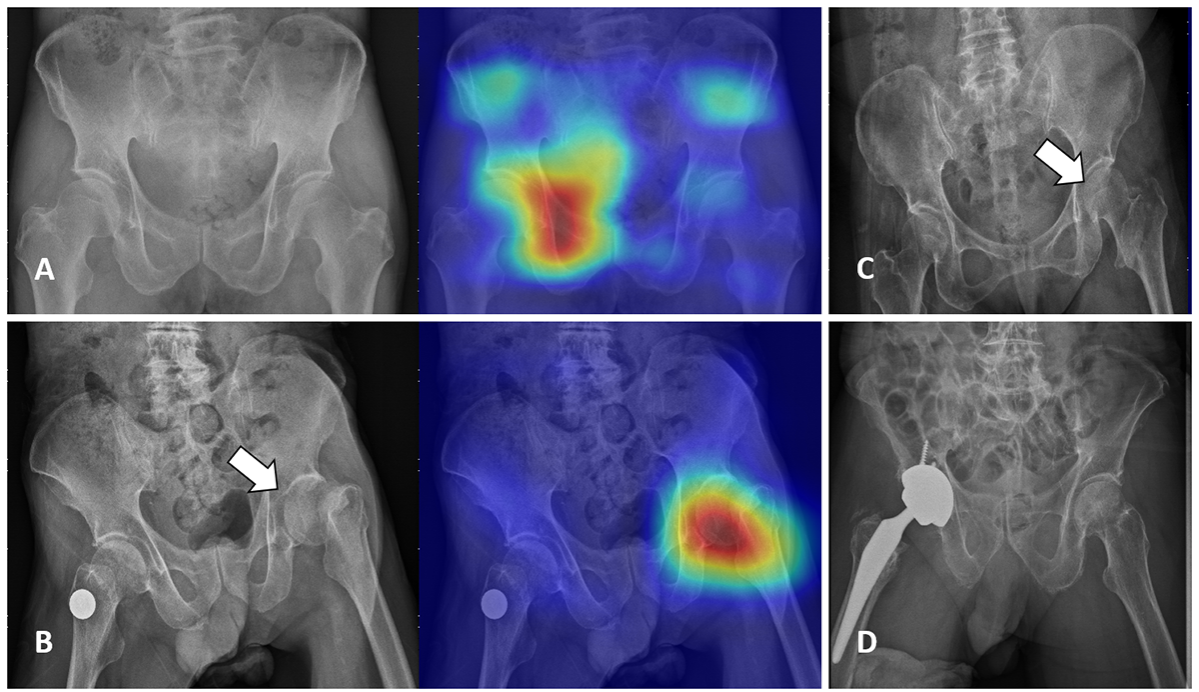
Examples of inconsistencies between the participants and the algorithm. (A) A pelvic x-ray (PXR) without hip fracture that was overdiagnosed by the algorithm. No participant overdiagnosed in the physician-alone test, and only 1 (2.9%) participant overdiagnosed in the human-algorithm integration (HAI) test. (B) A PXR with left hip fracture. In the physician-alone test, 12 (35.3%) participants missed this fracture. In the HAI test, only 1 (2.9%) participant missed this fracture. (C) A PXR with left hip fracture that was missed by the algorithm. In the physician-alone test, 4 (11.8%) participants missed this fracture. In the HAI test, 3 (8.8%) participants missed this fracture. (D) A PXR without hip fracture. In the physician-alone test, 18 (52.9%) participants overdiagnosed this image. In the HAI test, only 5 (14.7%) participants overdiagnosed this image.
